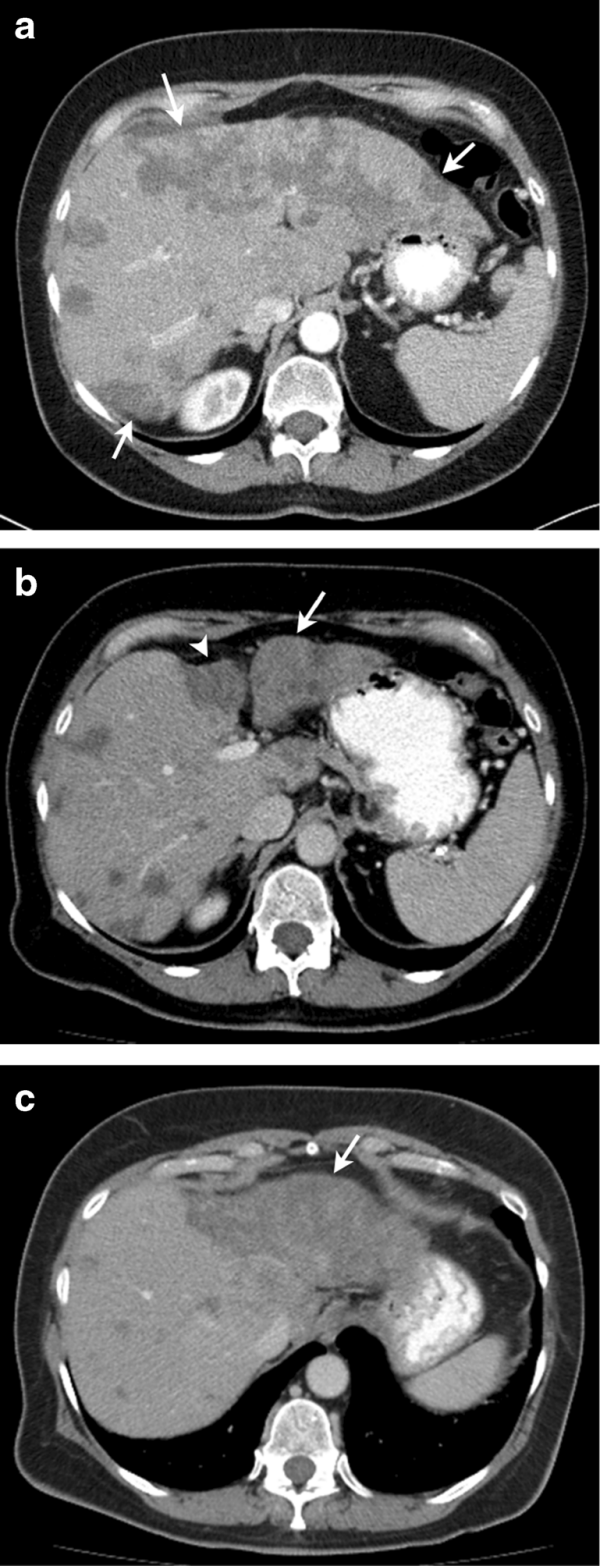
65-year-old woman with triple-positive breast cancer with multiple hepatic metastases treated with systemic chemotherapy. a Axial contrast-enhanced CT image before start of chemotherapy shows multiple low-attenuation hepatic metastatic lesions (*arrows*), more prominent in left lobe. b Axial contrast-enhanced CT image after 4 months of chemotherapy shows volume loss and surface nodularity in left lobe of liver (*arrow)*, with capsular retraction in segment IV (*arrowhead*) and decrease in size of metastatic lesions. c Axial contrast-enhanced CT image after 10 months of chemotherapy shows marked atrophy of left lobe of liver (*arrow*), with further decrease in size of metastatic lesions in right lobe
